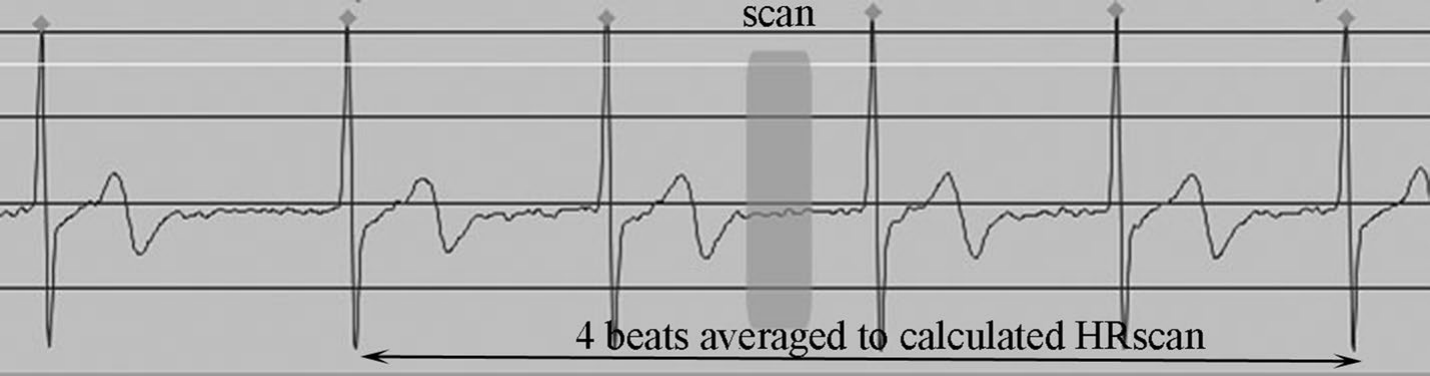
Definition of the four beats and the timing of exposure. HRscan was defined as the average HR of consecutive four beats (*arrow*), with the second beat corresponding to the first CT cardio angiogram scan. The scan duration took place during the RR interval within the *gray box*. The beginning of the scan was the left edge of the *gray box*, and the end of the scan was the right edge of the *gray box*
